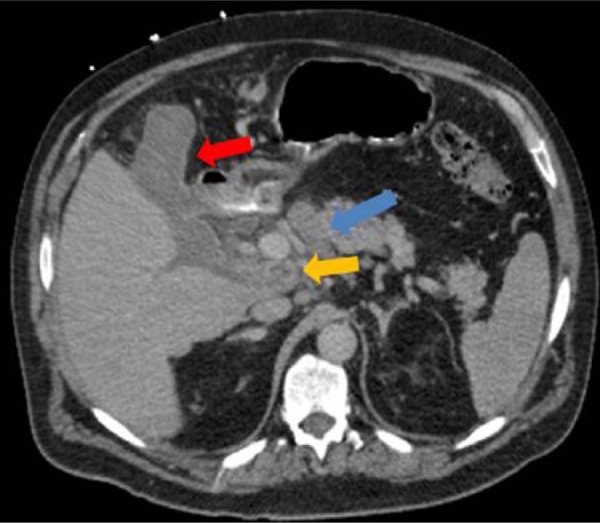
CT scan of the abdomen on presentation to our institution. Description: CT scan at the level of porta hepatis and pancreas showing high-density fluid collection/hemorrhage (red arrow) from the patient’s previous complicated biopsy. There is a mixed attenuated mass between the liver and pancreas (yellow arrow) and a well-defined lesion at the junction between the neck and body of pancreas which represents lymph node (blue arrow).
